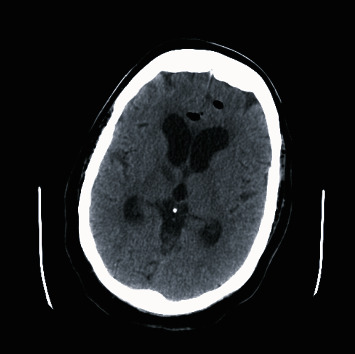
Brain CT demonstrating hydrocephalus and pneumocephalus.
